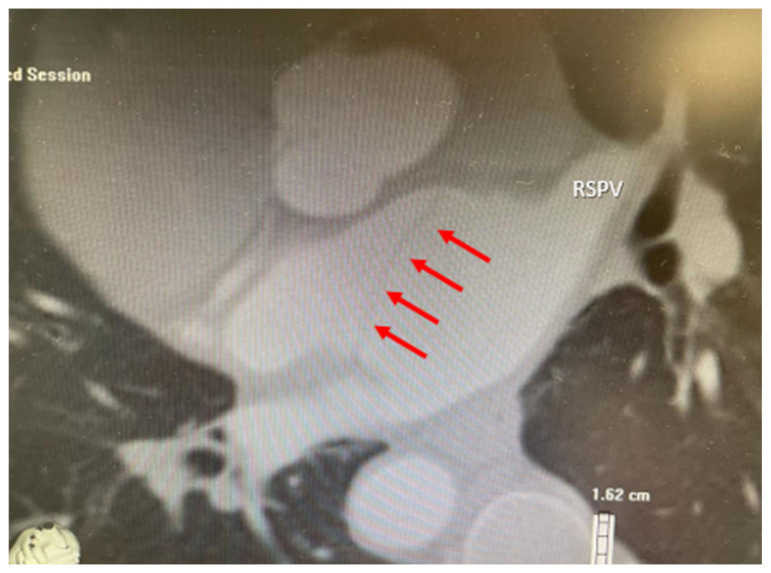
Multidetector computed tomography angiography confirming the presence of two separate left atrial chambers, the superoposterior atrial chamber which receives the pulmonary veins and the inferoanterior atrial chamber, separated by a membrane (red arrows). RSPV—right superior pulmonary vein.
